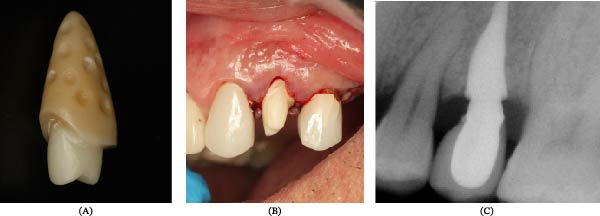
(A) Three‐dimensional CAD/CAM rendering of the milled root‐analog implant (RAI), generated from a high‐resolution intraoral scan of the extracted root; digital measurements were based on a millimeter‐calibrated design environment. (B) Clinical photographic view showing the insertion of the RAI following atraumatic extraction; soft tissue landmarks included for spatial reference. (C) Periapical radiograph obtained using a standardized long‐cone paralleling technique; radiographic scale calibrated in millimeters for assessment of implant position and crestal bone alignment.
